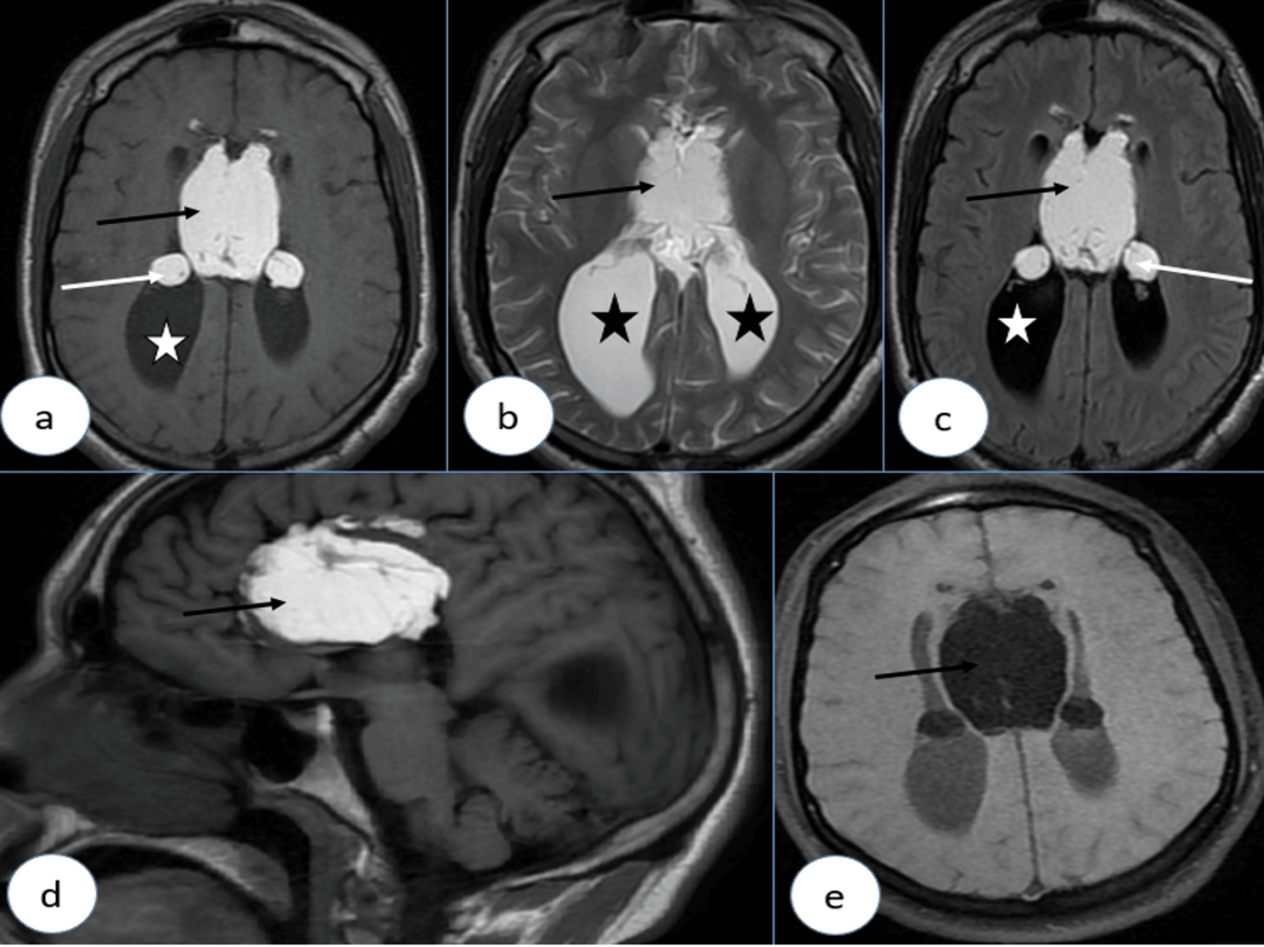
Brain MRI with axial T1 FSE (A), axial T2 (B), axial T2 FLAIR (C), sagittal T1 FSE (D) and axial T1 Fat Sat (E), showing a tubulonodular pericallosal lipoma (black arrows), appearing hyperintense on T1 and T2, with signal loss on the T1 Fat Saturation sequence, associated with a agenesis of the splenium of the corpus callosum and colpocephaly (stars). Intraventricular extension through the foramina of Monro (white arrows) is also noted MRI: magnetic resonance imaging (MRI); T1 FSE: fast spin echo; T1 Fat Sat: MRI T1 fat saturated; FLAIR: fluid-attenuated inversion recovery
